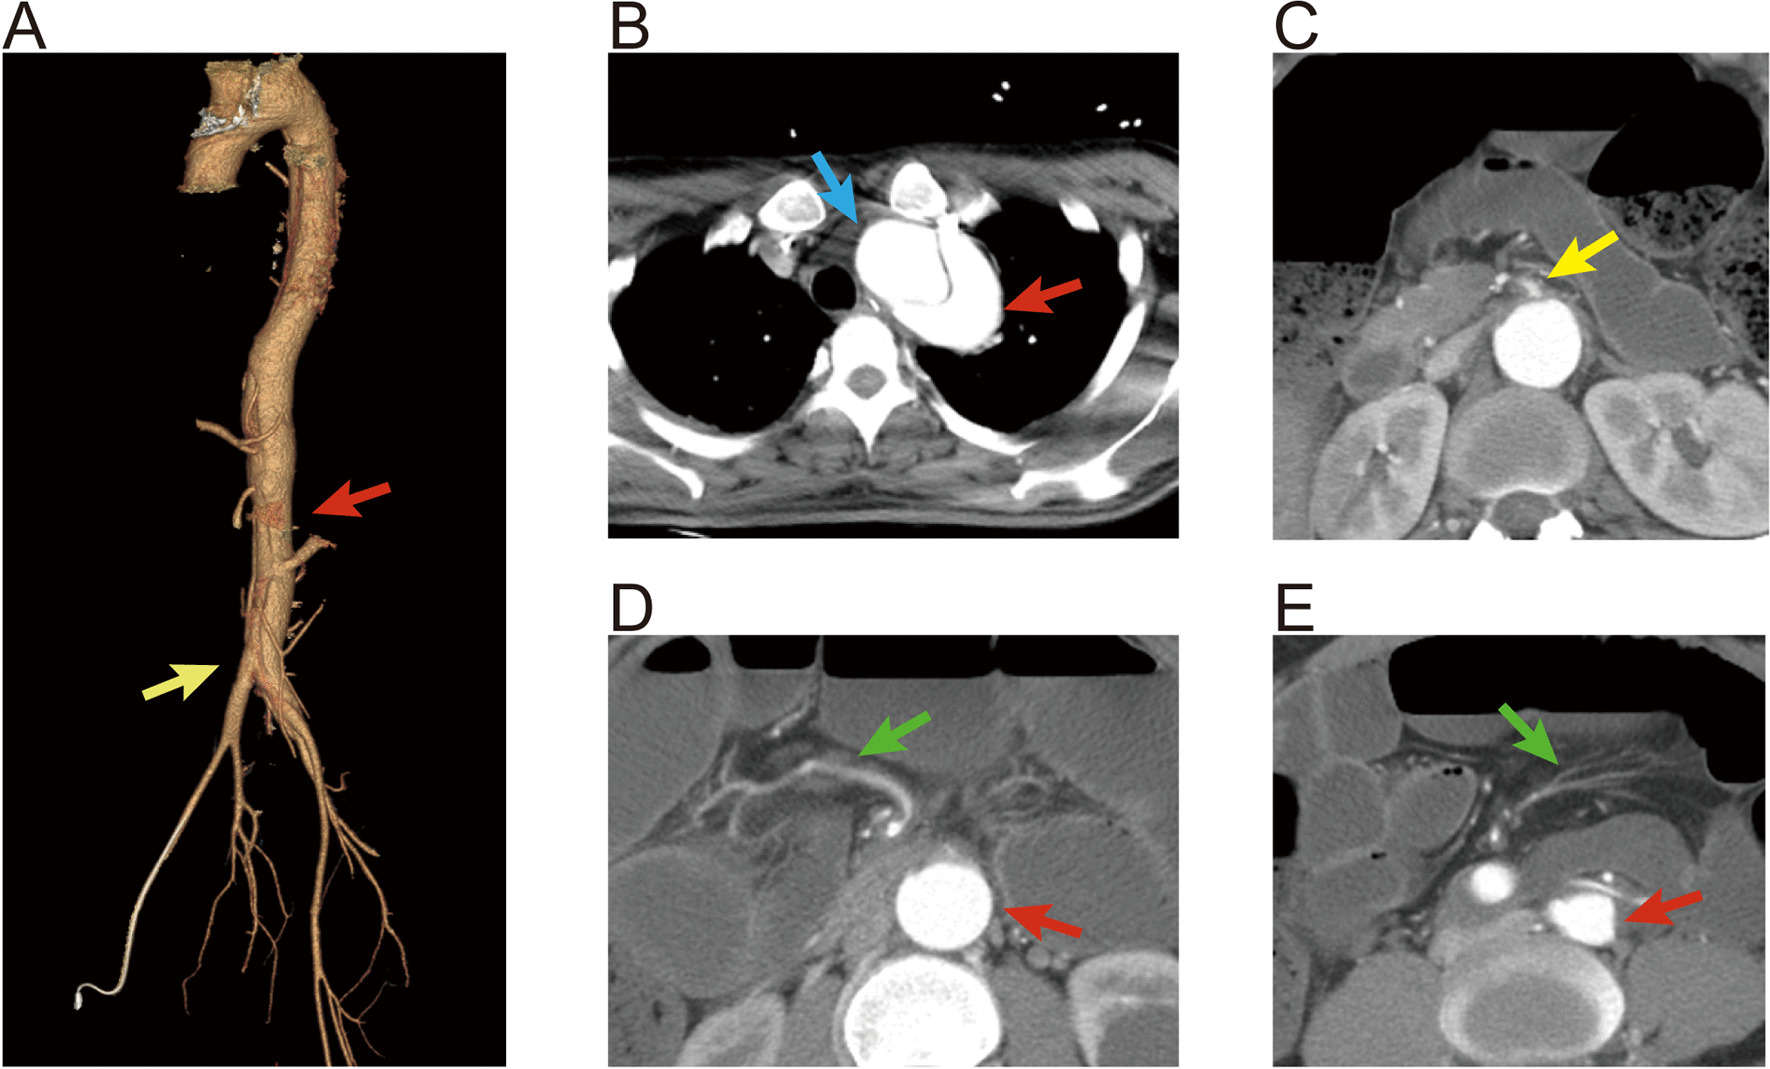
Thoracic and abdominal CTA of the patient showed type B aortic dissection (the true lumen and the false lumen of abdominal aorta were respectively marked with red and blue arrows, B) with a coarctated lumen of the initial segment of the superior mesenteric artery (yellow arrows, A and C) and a noticeable vessel tree in the enhanced sequence (green arrows, D and E)
